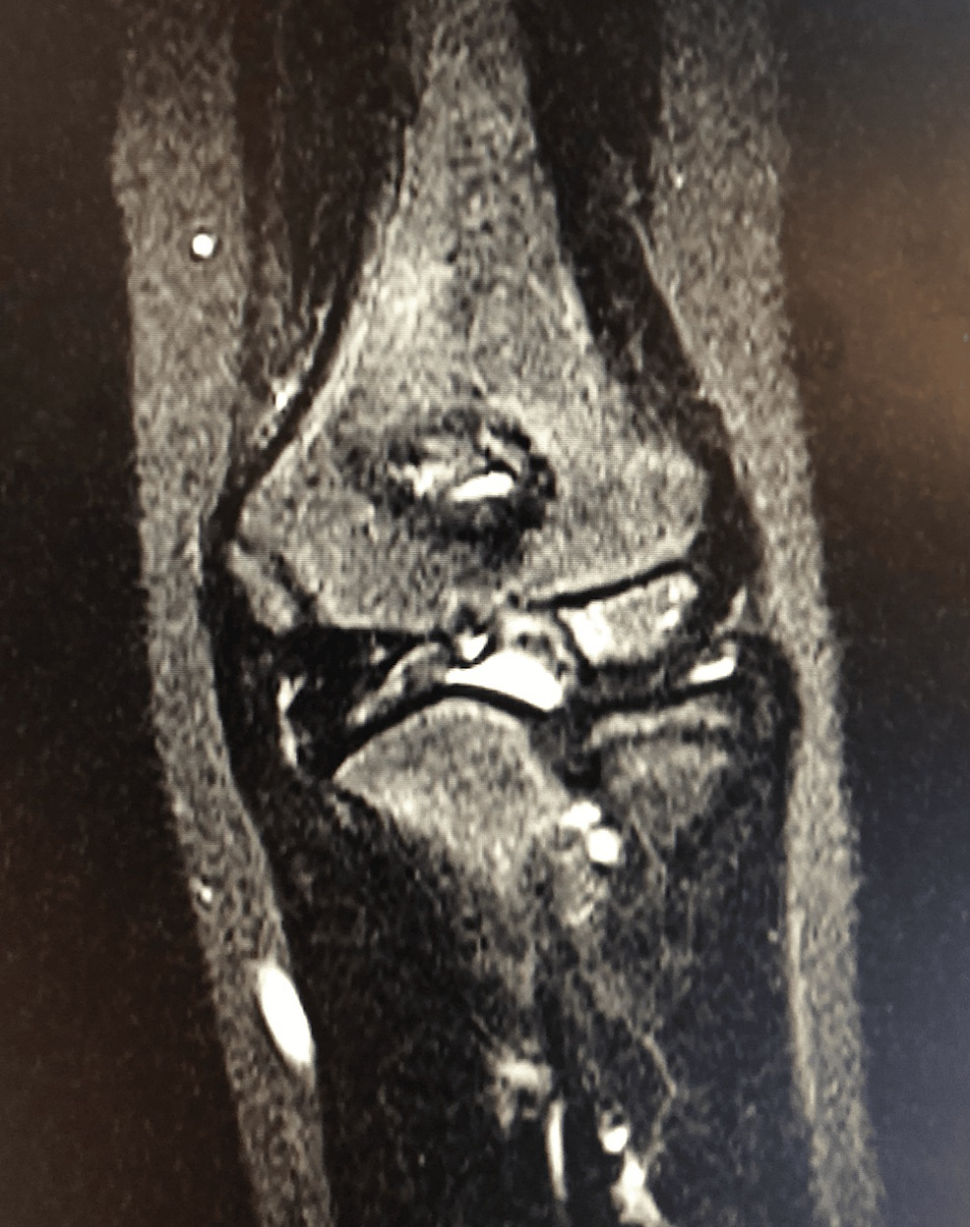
STIR coronal section MRI of the elbow joint after the second injury. Hydrarthrosis and deformity of the lateral part of the trochlea are apparent. STIR: Short tau inversion recovery.
